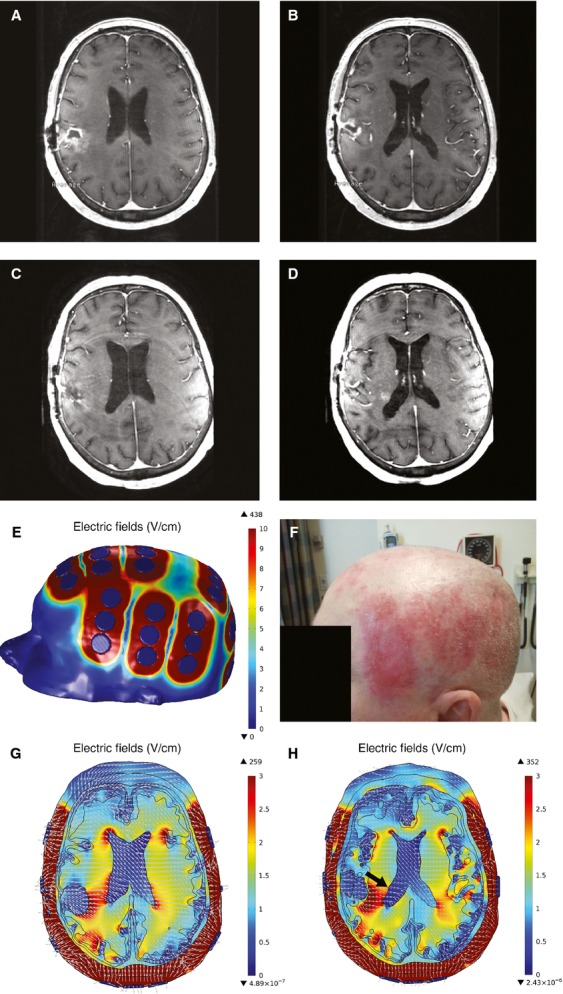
Tumor treating fields (TTFields) therapy for recurrent glioblastoma. Recurrent glioblastoma in the posterior right frontal brain was detected in a 67-year-old woman 6 months after initial treatment. The tumor can be seen in two successive slices, upper slice (A) and lower slice (B) on gadolinium-enhanced T1-weighted MP RAGE sequence. Her recurrent disease was then treated with bevacizumab and TTFields using the NovoTTF-100A device and after 24 months a new site of disease was detected at the lateral border of the right lateral ventricle (D), while the primary tumor was stable (C). Computed modeling revealed that the electric field strength was highest around the ceramic disks forming each array (E), which is also located at the same site of scalp irritation experienced by the patient (F). High field strength is also seen at the ventricular horns and there is inhomogeneous electric field distribution on the tumor (G). The new enhancing tumor is situated at the lateral border of the right lateral ventricle in an area with relatively lower electric field strength (H, arrow).
